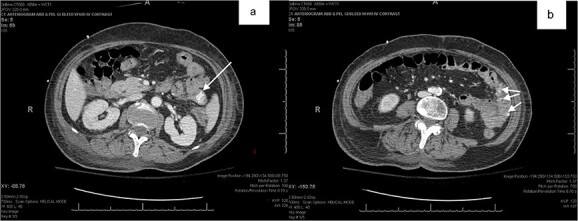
(a,b) abdominal CT with oral contrast identifying extravasation in the jejunal loop.
